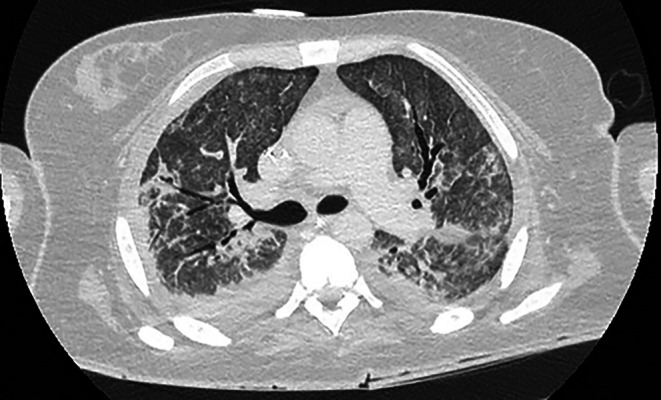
CT chest (lung window) 24 h following insertion of bilateral EBVs showing improvement in aeration.
